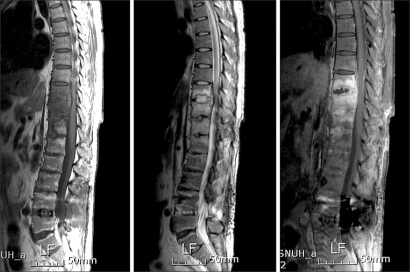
Sagittal T1- and T2-weighted and contrast-enhanced images at 22 months postoperative. MRI revealed progression of the bone marrow signal change, with enhancement of the lesion at T10-L1 and increased size of the anterior epidural abscess at T10-T12.
